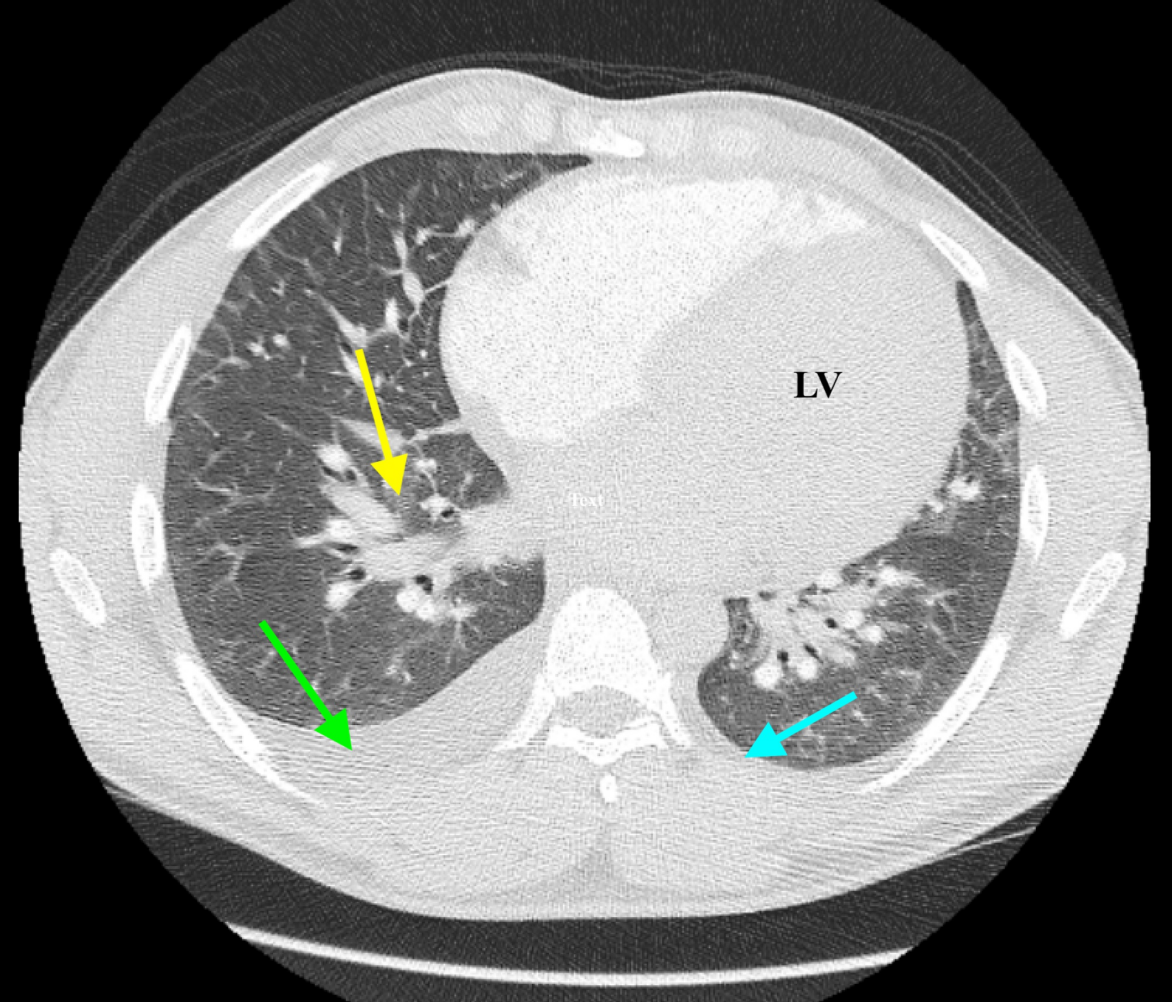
CTA of the chest demonstrating mild-to-moderate R pleural effusion (green arrow), small left pleural effusion (blue arrow), pulmonary vascular congestion consistent with pulmonary edema (yellow arrow), and enlarged heart with dilated LV. Obstructive lung disease changes were not visualized. CTA, computed tomography angiography; LV, left ventricle
